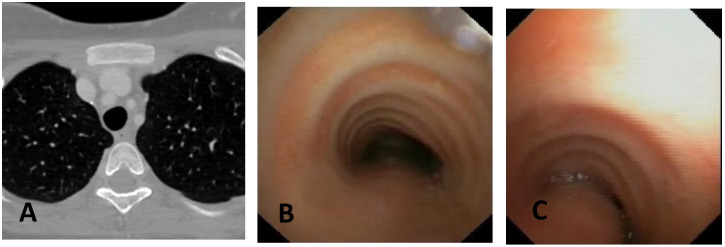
(A) Chest CT with CM: Slight tracheal compression from IA. (B) SVBS: Well-preserved tracheal lumen up to junction between the middle and distal third, where clear pulsating extrinsic compression from IA deforms the trachea. (C) DVBS: when the patient performs abdominal straining, the hypermobile pars membranacea completely occludes the trachea at the level of extrinsic compression. The presence of granulomas at this level proves this contact occurs recurrently. Abbreviations. CT: Computed Tomography; CM: Contrast Medium; SVBS: Static Videobronchoscopy; IA: Innominate Artery; DVBS: Dynamic Videobronchoscopy.
